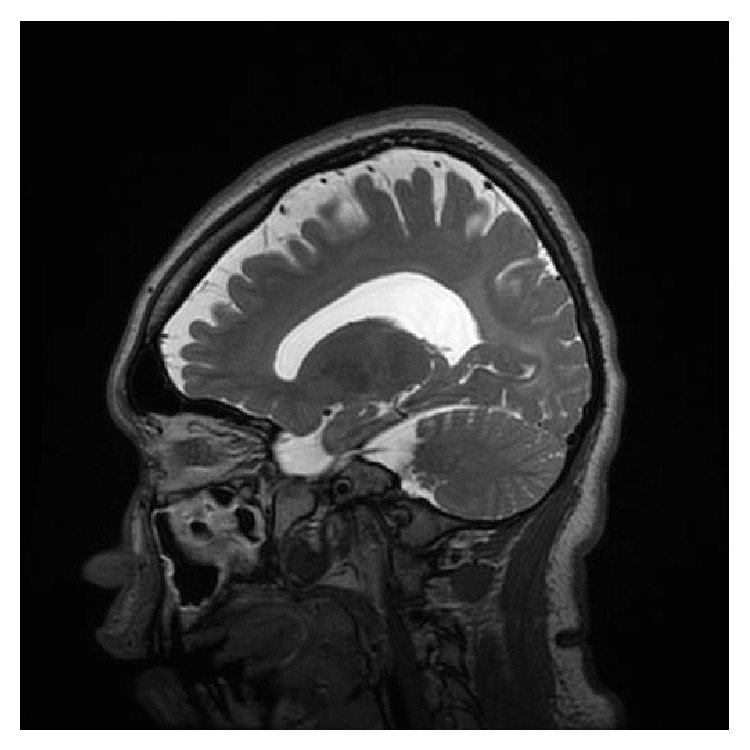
Sagittal MRI view of the brain at the age of 17 showing diffuse increased T2 signal in the subcortical and periventricular white matter demonstrating diffuse leukodystrophy. GE sagittal T2 CUBE image performed on a 3T MR GE scanner with TR: 3000, TE: 69.1.
